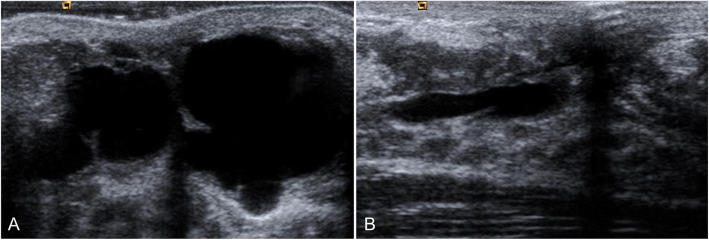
Retroareolar cyst in an adolescent. A 15-year-old girl presented with a palpable retroareolar mass in her left breast. Ultrasound shows a large thin-walled cystic lesion (a). Follow-up ultrasound 6 months later shows a marked decrease in the size of the lesion, which is oval and elongated (b)
